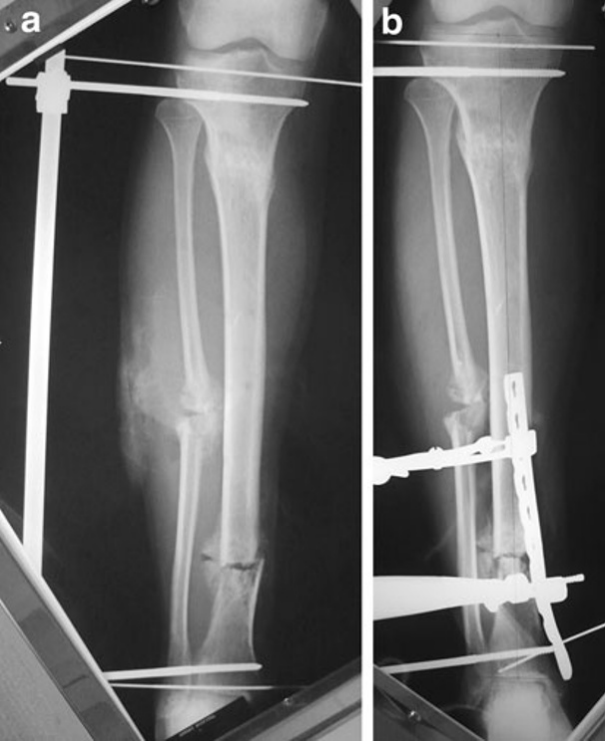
a, b intra-operative radiographs after deformity correction and preliminary plate positioning
